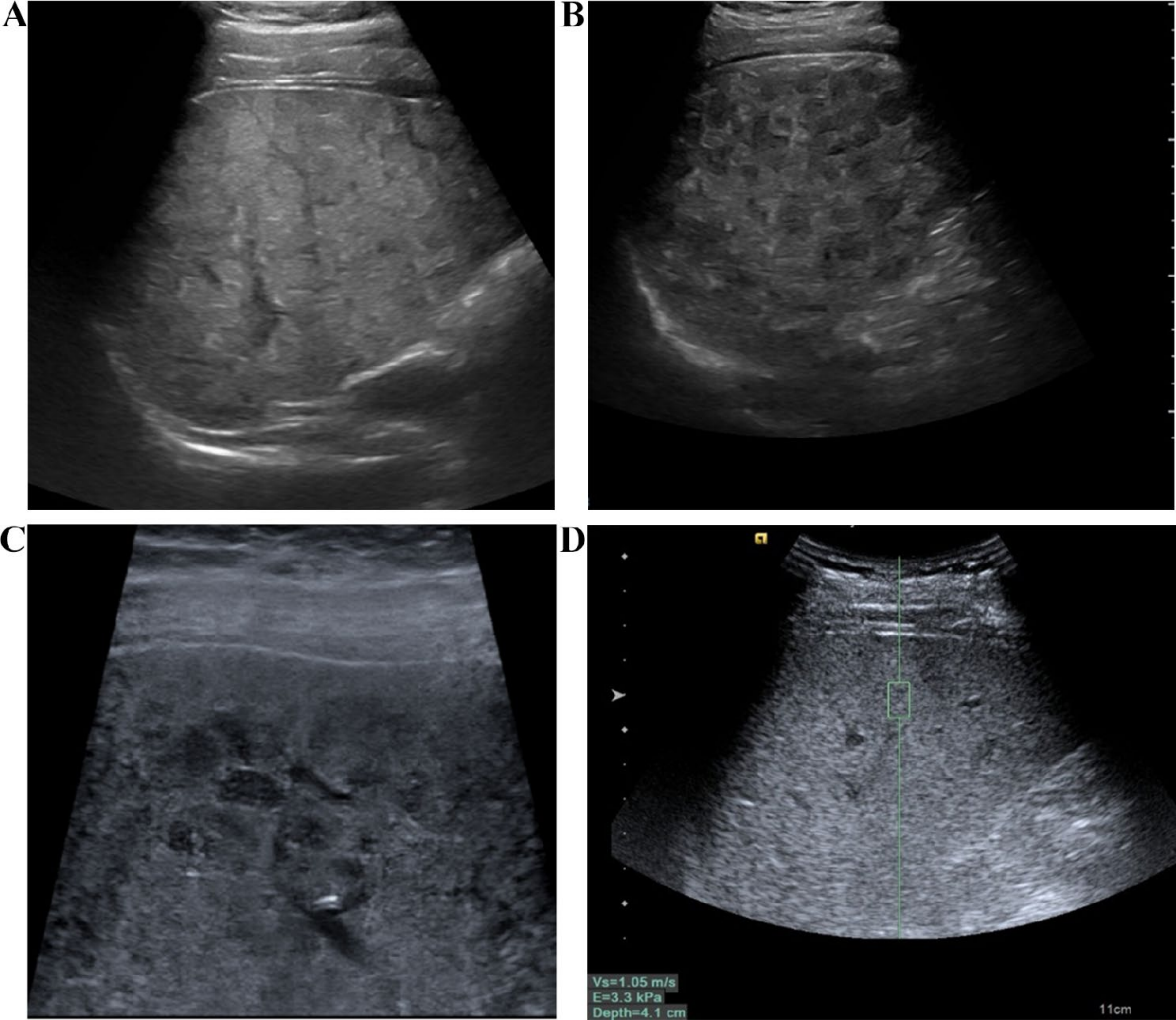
The examination of conventional ultrasound and ARFI. A The liver of patients with WD exhibited a patchy, fatty appearance. B Scattered nodular hypoechoic regions were observed in the liver of WD patients. C The liver of WD patients displayed honeycomb-like nodules when observed with a linear array probe, while the liver capsule appeared smooth. D Liver stiffness was assessed using ARFI technology
